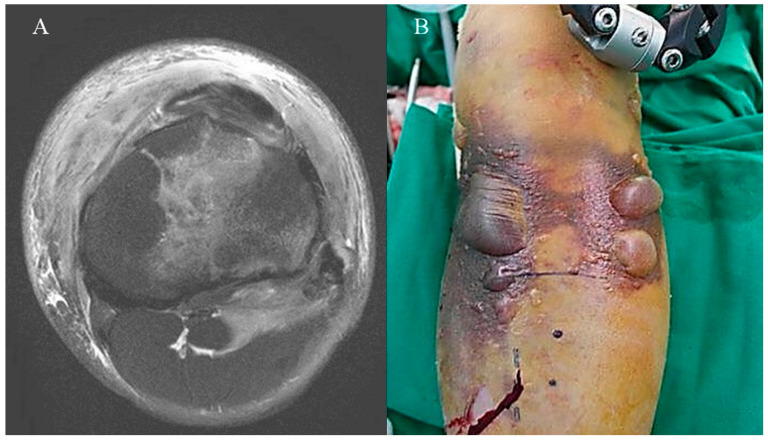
Same patient with Figure 2 (A) Preoperative MRI shows soft tissue injury of the Tscherne classification grade 3. (B) On day 1 after injury, the patient’s lower leg was severely swollen and showed hemorrhagic fracture blisters.
